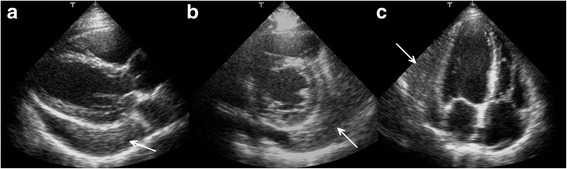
Transthoracic 2D echocardiography in the parasternal long-axis plane (panel a), short-axis plane (panel b) and apical four-chamber view (panel c). *Arrows* show the intrapericardial mass
